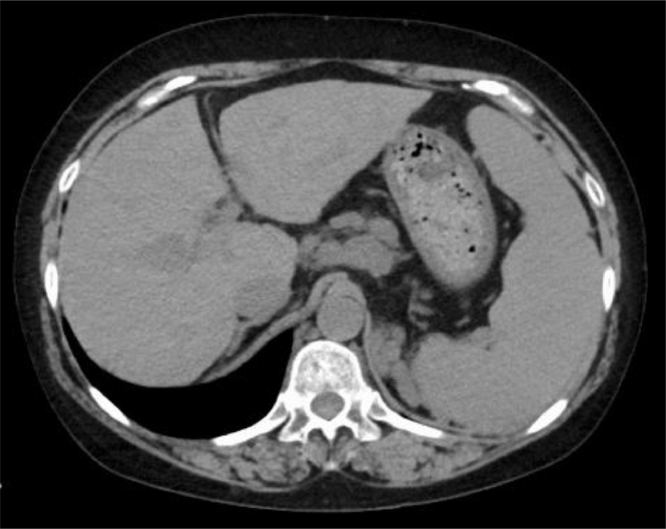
Computed tomography findings showing hepatic morphological changes and splenomegaly.
